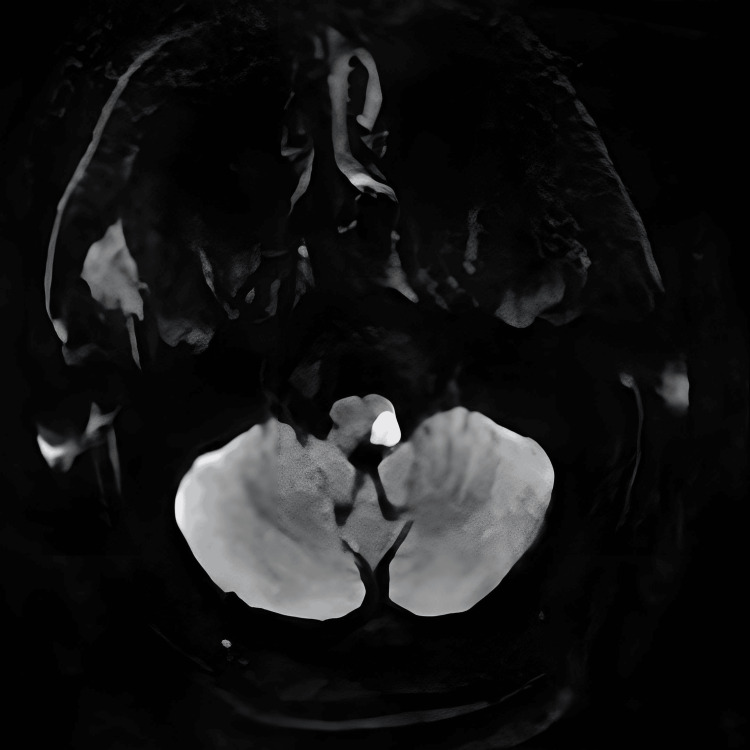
Diffusion-weighted axial MRI showing restricted diffusion involving the left medullary region, consistent with a subacute left lateral medullary infarct
